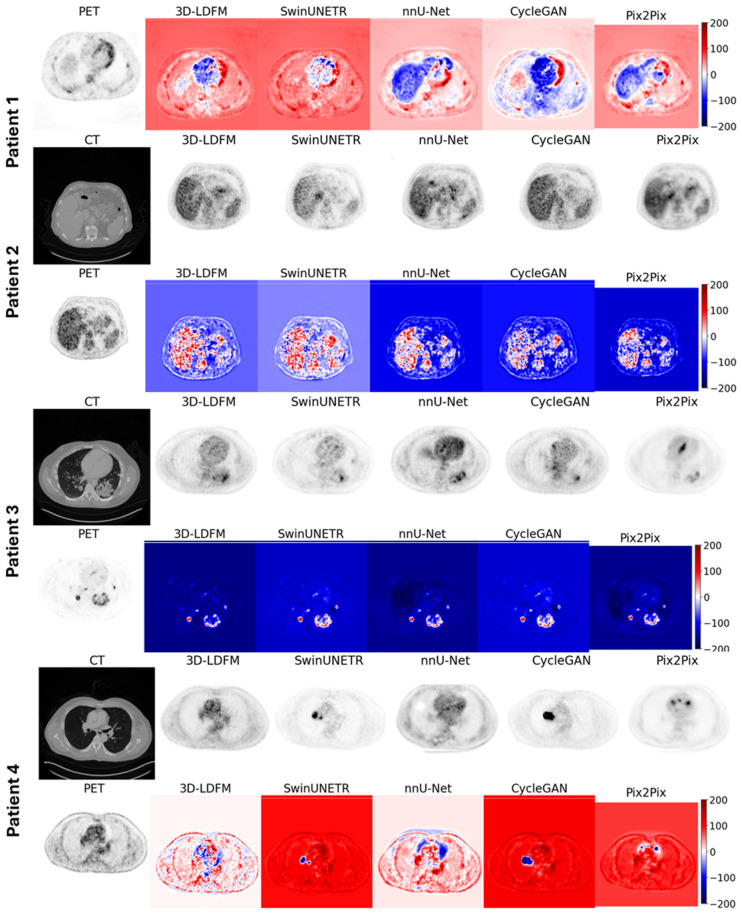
Qualitative comparison of synthetic PET generation in the lung-to-head region. Representative test cases are shown with the input CT, reference PET, and synthetic PET outputs from each method.
